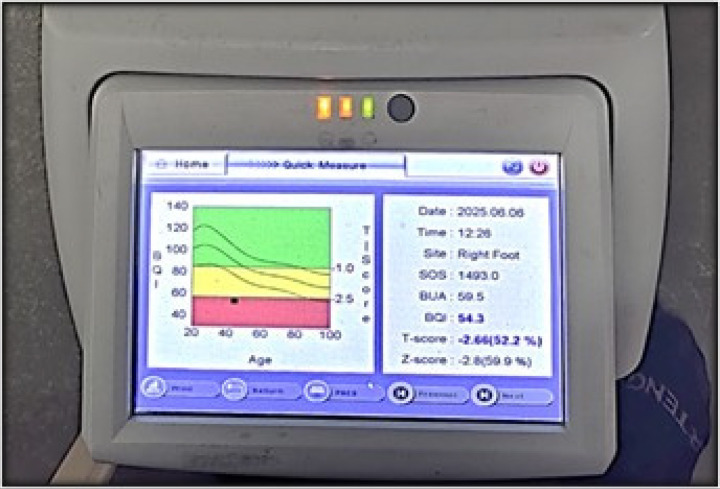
Acquisition of T-score using the calcaneus quantitative ultrasound machine.
